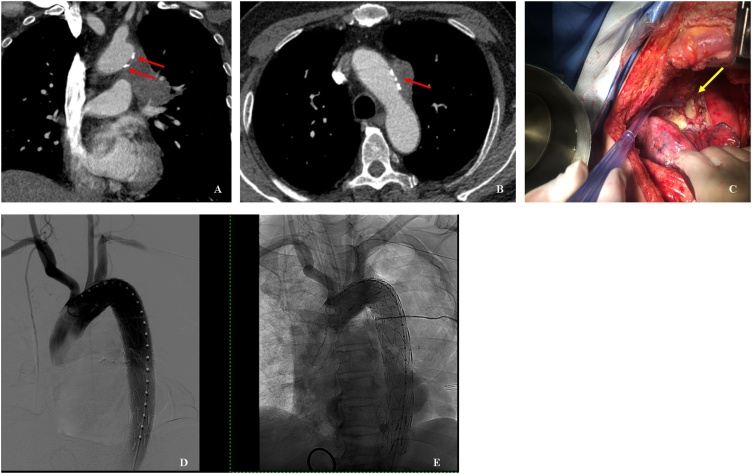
A and B. CT scan showing the left upper lobe tumor mass infiltrating the main left pulmonary artery and the bronchus. Fig. 1C. Red arrows highlighting the position of the atherosclerotic plaque. Fig. 1D and E. Revealing the endograft stent placed in the aorta by the interventional radiologist through the femoral artery. CT: computer tomography.
